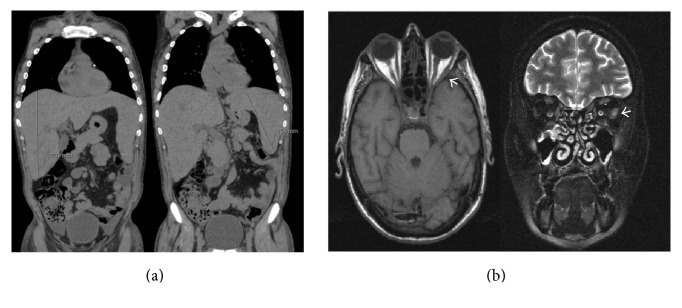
Thoracic-abdominal-pelvic computerized tomography scan showing the hepatosplenomegaly (liver and spleen longitudinal axis of 209 cm and 158 cm, resp.), without other significant abnormalities (a). Cerebral MR images showing swelling of the left lateral rectus muscle on a T2-weighted image, with no other additional findings (b).
